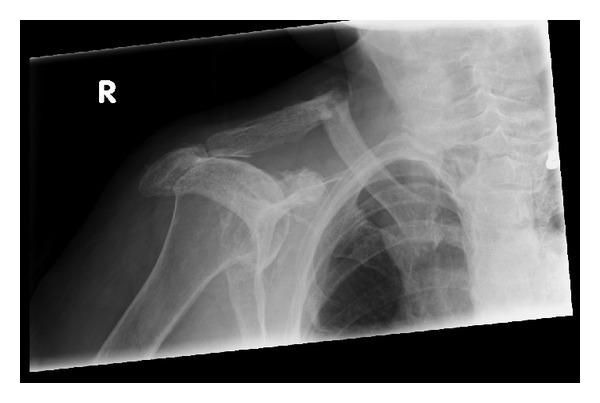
Follow-up radiograph at fracture clinic, four weeks following conservative management, demonstrating signs of callus formation of the right mid-clavicular transverse fracture. Superior cortical thickening and a small spike of the fracture fragments are also seen in this figure.
